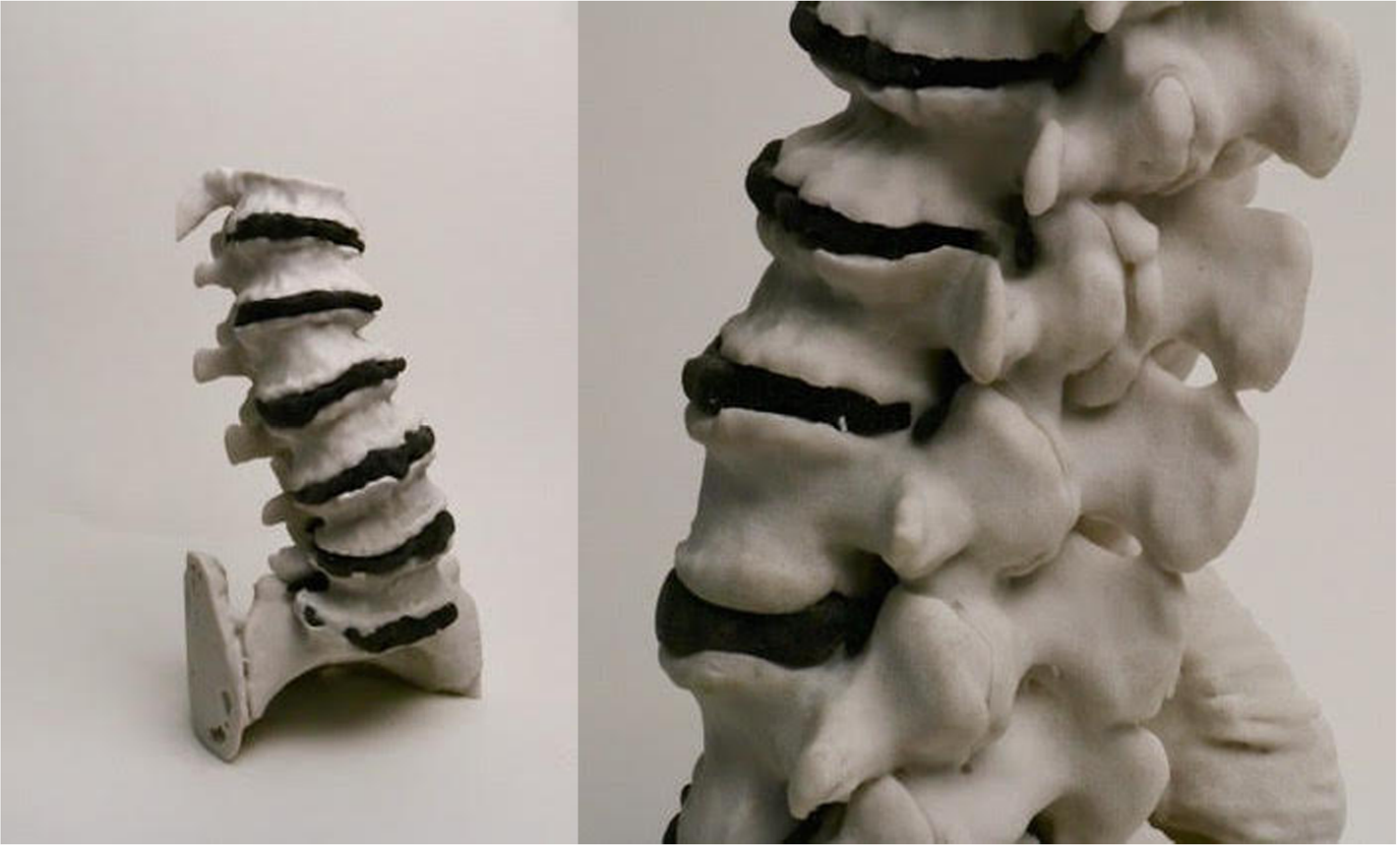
3D printed model of a lumbosacral spine with realistic spondylosis, including levoconvex scoliosis and degenerative facet arthropathy, modeled from a real patient CT. Ivory-colored vertebral bodies were printed using calcium sulfate hemihydrate. Black-colored intervertebral discs were printed using Stratasys Polyjet FLX980 Tango Blacrk
